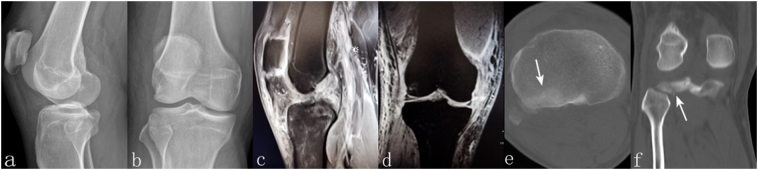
The patella alta was observed from lateral view of radiology, and there was no finding of obvious bony lesion (a, b). The ruptures of the PT, ACL, and MCL were confirmed by the MRI images (c, d). Depression and split of the posterolateral tibial plateau (white arrow) were noted on transverse and sagittal cuts of the CT scan (e, f).
